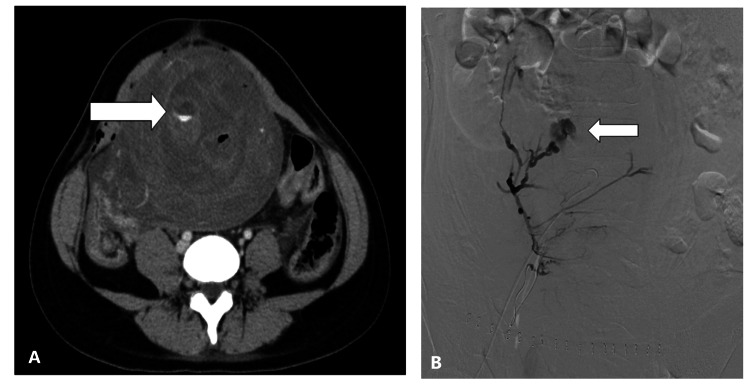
Radiologic images of a 25-year-old woman with bleeding within 24 hours after vaginal delivery. (A) A contrast-enhanced CT image shows active contrast extravasation in the uterus (arrow). (B) A right uterine arteriogram shows active contrast extravasation from the right uterine artery (arrow).
